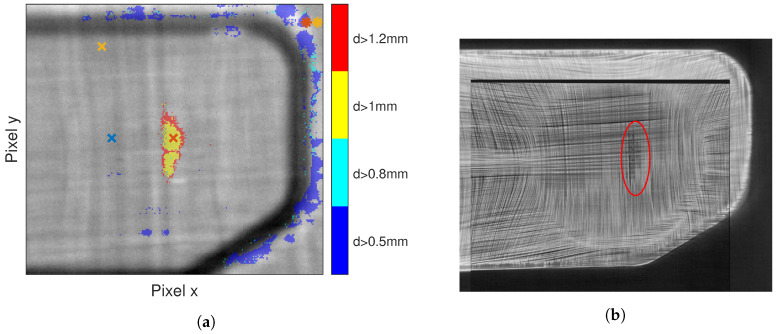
Comparison of active thermography with 3D-computed tomography: (a) results of the proposed algorithm and (b) the density map obtained by 3D-XCT at a depth of 1 mm from the front side.
